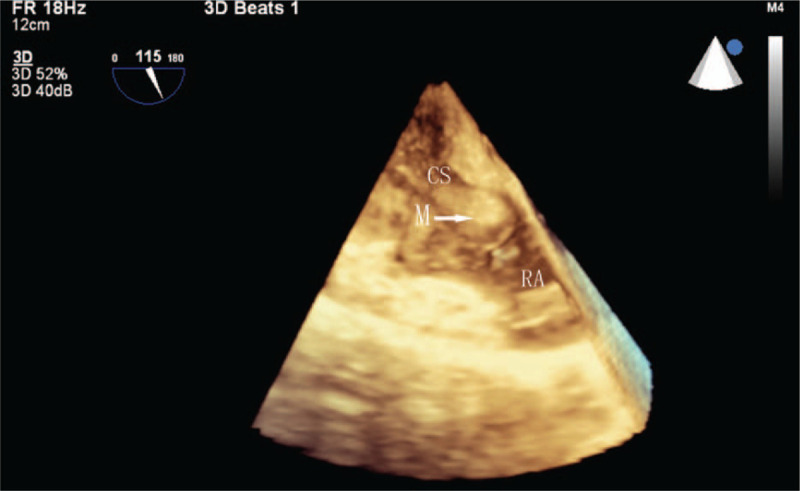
Three-dimensional transesophageal echocardiography showed the morphology of the mass. CS = coronary sinus, M = mass, RA = right atrium.
